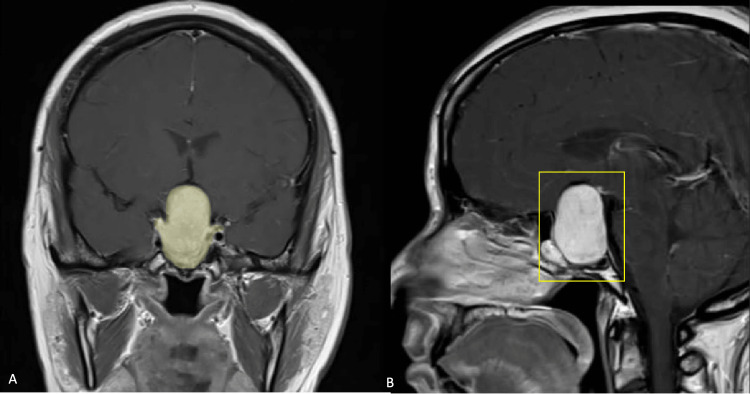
C+ MRI in coronal (A) and sagittal (B) projections showing a pituitary marcroadenoma (yellow shading) of Hardy-Wilson 2C, Knosp II classification
